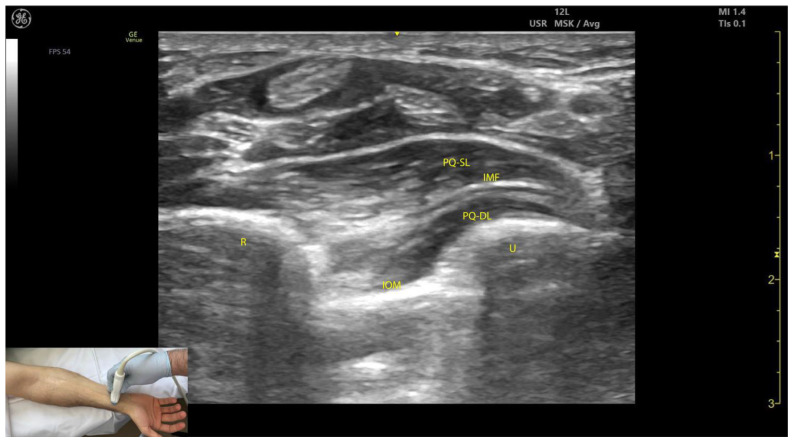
Ultrasound anatomy of the pronator quadratus (PQ) with key landmarks: PQ-SL—pronator quadratus superficial layer; PQ-DL—pronator quadratus deep layer; IOM—interosseous membrane; IMF—intramuscular fascia.
